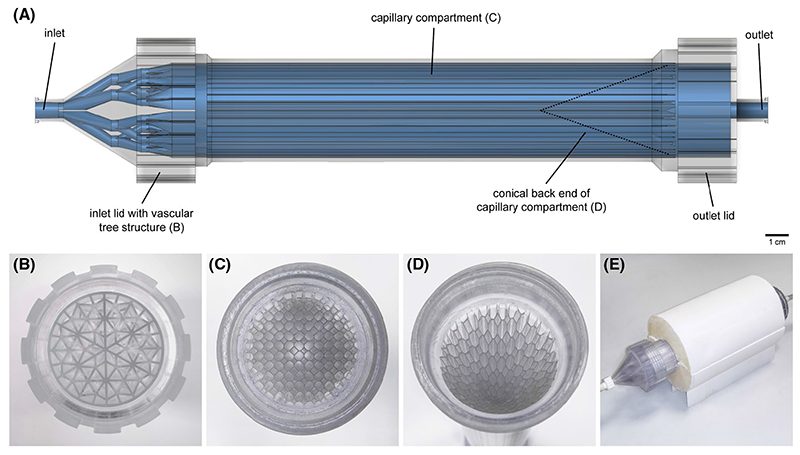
The geometry of the synthetic myocardium. Image (A) is a 3D rendering of the fully assembled synthetic myocardium showing the printed parts (transparent grey) and the internal contrast dispersion volume (blue). Photos show the inlet lid with the vascular tree structure (B), the front end of the capillary compartment (C), and the back end of the capillary compartment with the conical taper applying a 30% transmural difference in capillary length (D). The myocardium is placed on a water-filled cylindrical base providing signal for MRI data normalization (E)
